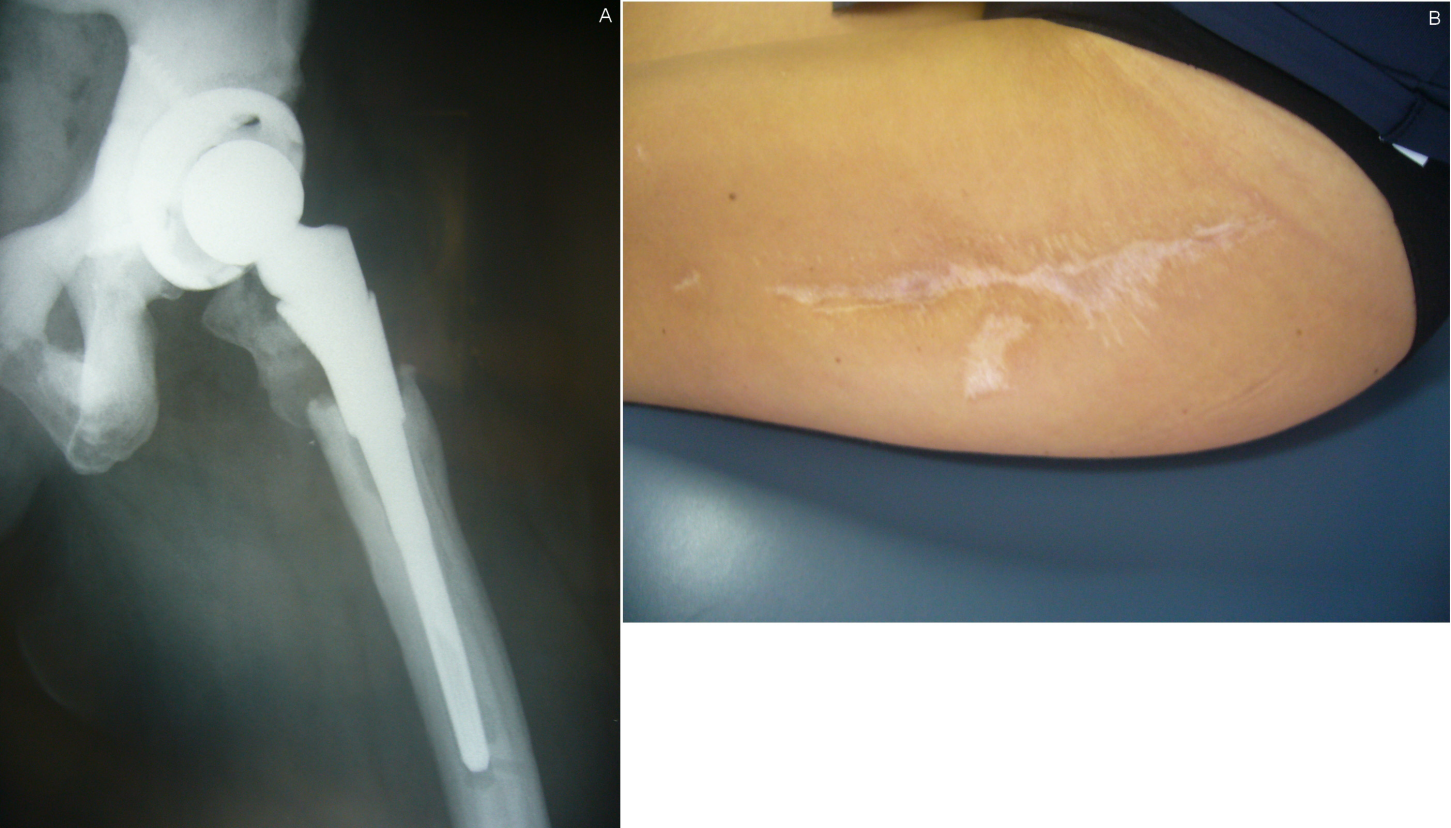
X-ray (A) and clinical picture two years after surgery.
